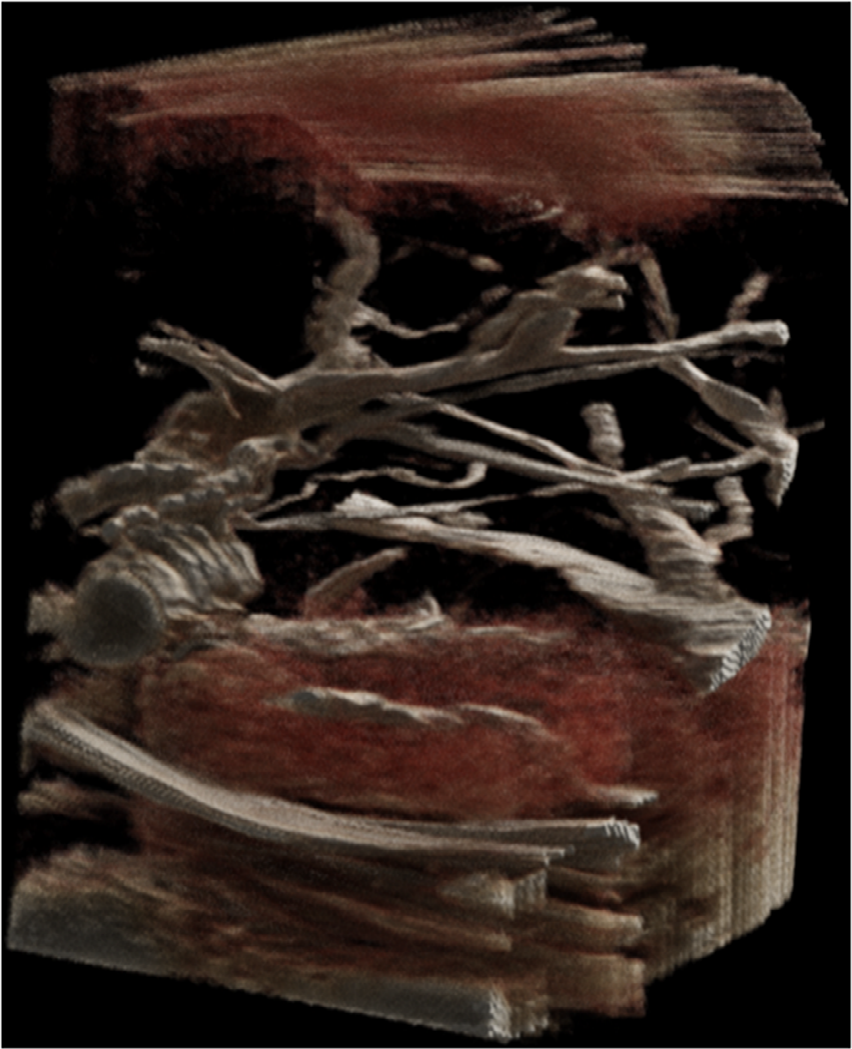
Example of visualization of an ultrasound three‐dimensional (3D) reconstruction using the Siemens Healthineers Cinematic renderer.
